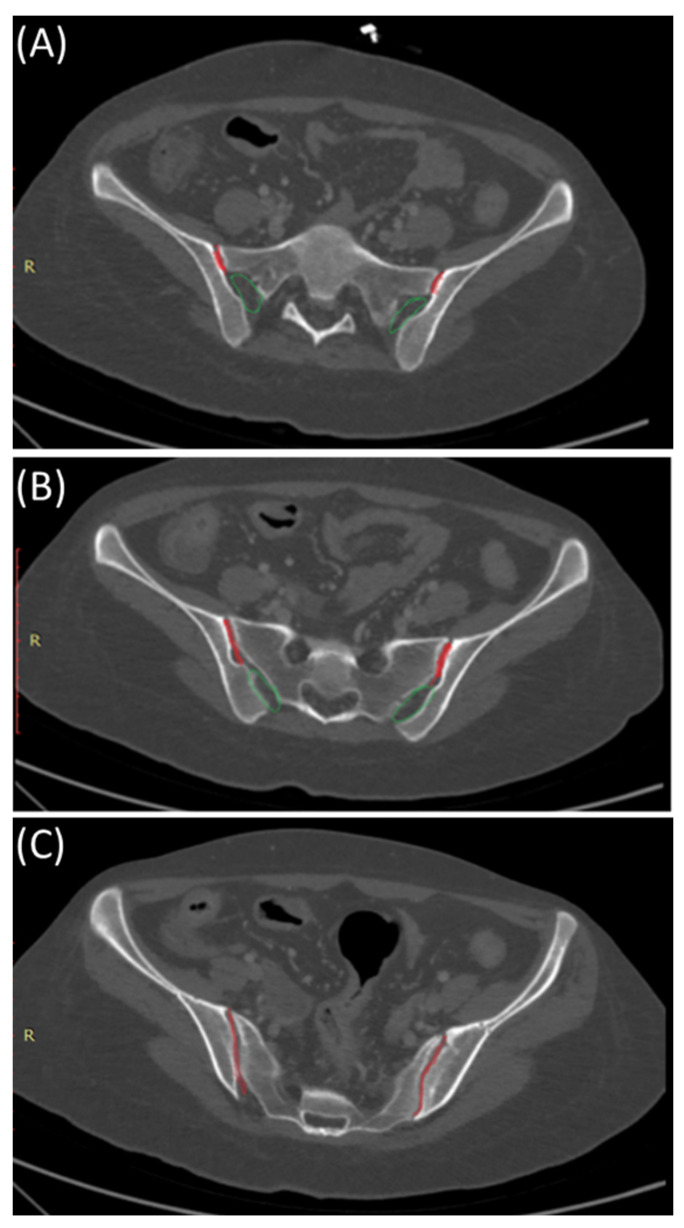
Sacroiliac joint anatomy: Synovial and syndesmotic parts. Axial bone window CT images indicating the position of the synovial (red) and ligamentous (green) components of the sacroiliac joint from cranial (A), mid- (B), and caudal (C) parts of the sacroiliac joints.
